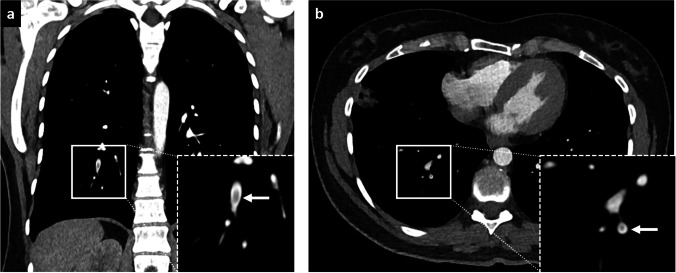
Low-dose PCD-CT demonstrating peripheral pulmonary embolism at segment level in the right lower lobe at coronal (a) and corresponding axial (b) 60-keV reconstructions. The minute contrast medium filling defect is magnified and highlighted by arrows. *PCD-CT*, *photon-counting detector CT*
